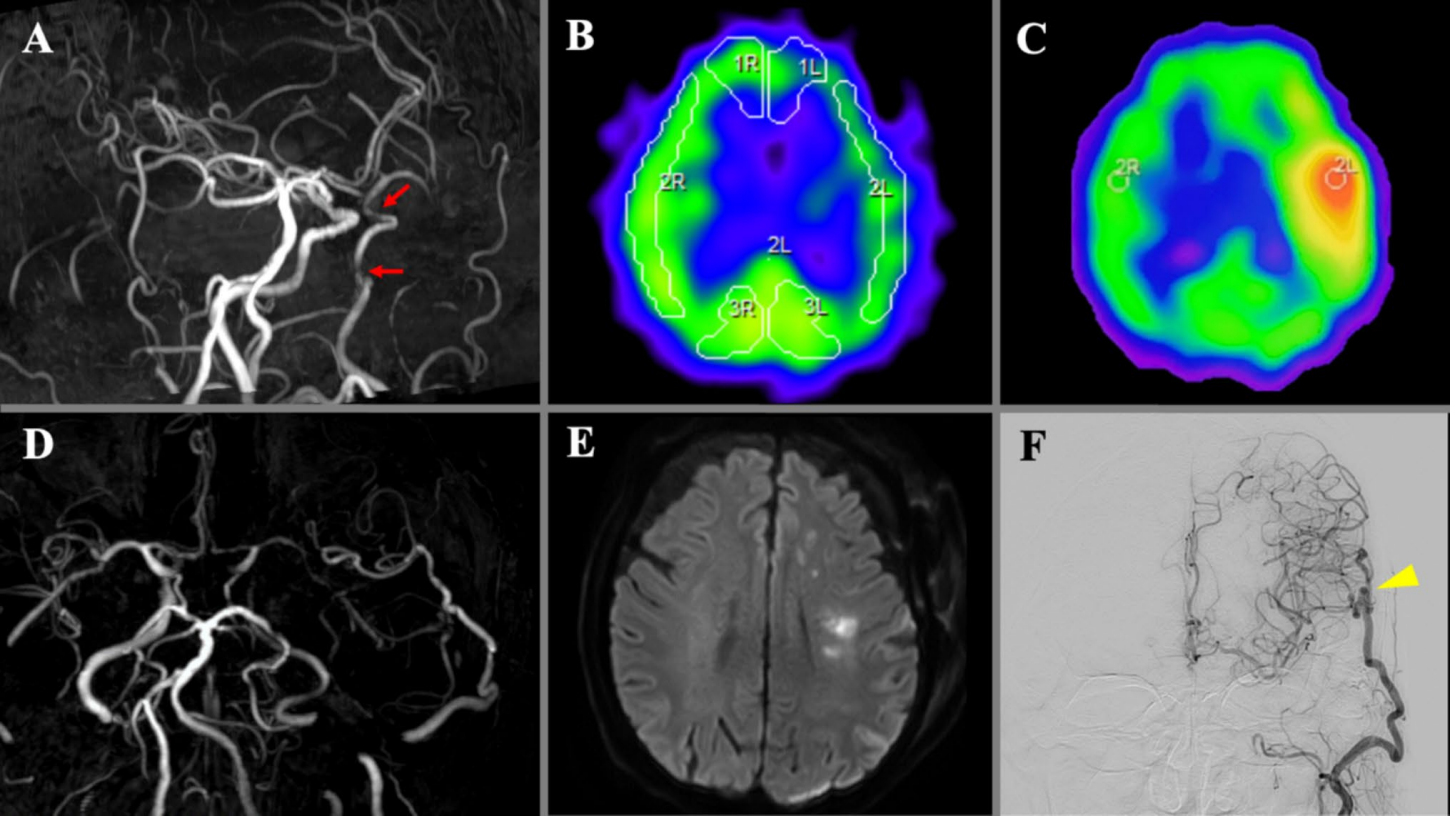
A Preoperative MRA shows a diminished flow signal in the left ICA due to tandem stenoses (red arrows). B Preoperative SPECT demonstrates markedly reduced cerebral blood flow (CBF) and cerebrovascular reactivity in the left anterior circulation. C Postoperative SPECT reveals focal hyperperfusion, with maximum CBF reaching 250% compared to the contralateral side. D, E On postoperative day 3, MRA (D) confirms good bypass patency, while diffusion-weighted imaging (E) shows a new infarction in the left corona radiata. F Postoperative left carotid angiography demonstrates adequate bypass-mediated perfusion of the entire anterior circulation, despite loss of anterograde flow in the left ICA
